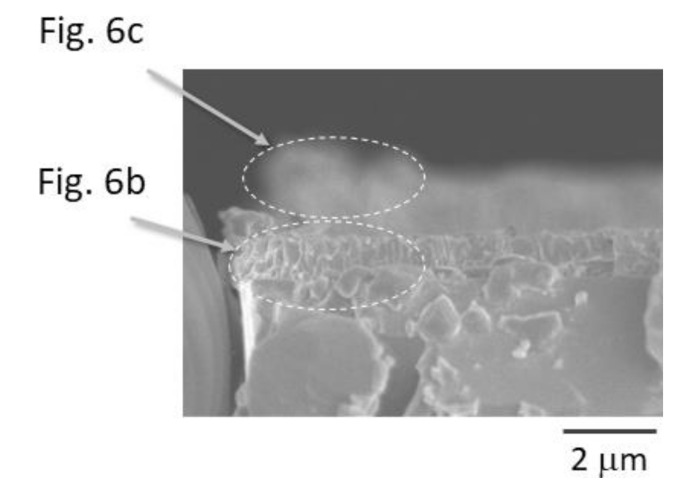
Side view of the X-ray-scanned sample by SEM.
